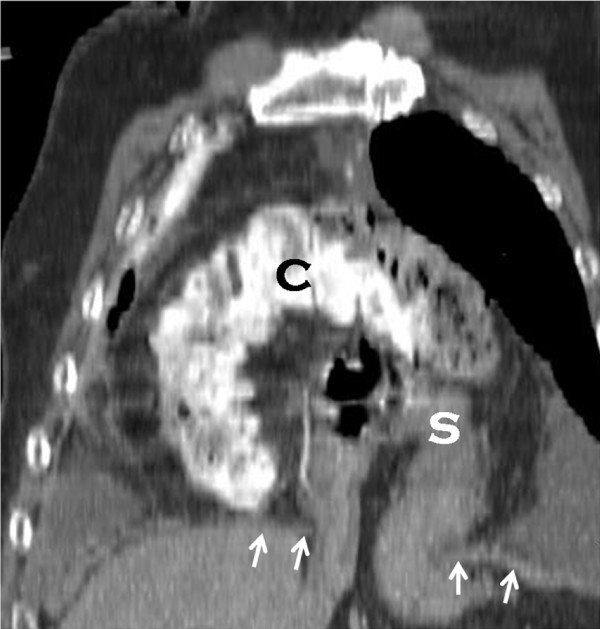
Chest Computed Tomography Scan. Sagittal oblique multiplanar reformat (MPR) from outside CT scan demonstrating discontinuity of diaphragm (white arrows) through which portions of the stomach (S) and transverse colon (C) have herniated.
